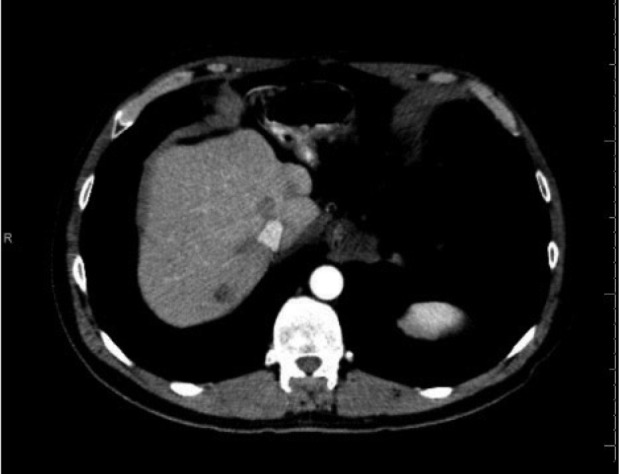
September 2011 CT scan of the chest, abdomen, and pelvis reveals an enlargement of a previously demonstrated segment 7 hepatic lesion.
